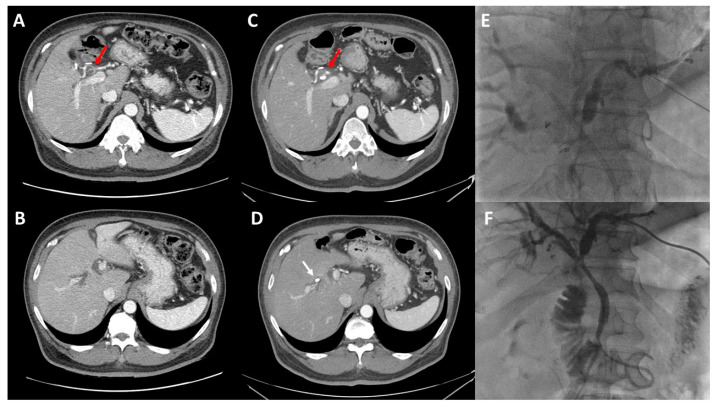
Repeat drainage following misplaced endoscopic biliary drain. Computed tomography showing perihilar tumor with involvement of right hepatic artery ((A), red arrow) with dilation of both left and right intrahepatic biliary trees (B). Repeat scan after placement of endoscopic biliary stent shows persistent arterial involvement ((C), red arrow) with inappropriate placement of the endoscopic stent into the right liver ((D), white arrow). The left liver (future liver remnant) remains undrained. Patient subsequently underwent selective percutaneous access of the left biliary tree (E) with placement of an internal-external biliary drain (F).
